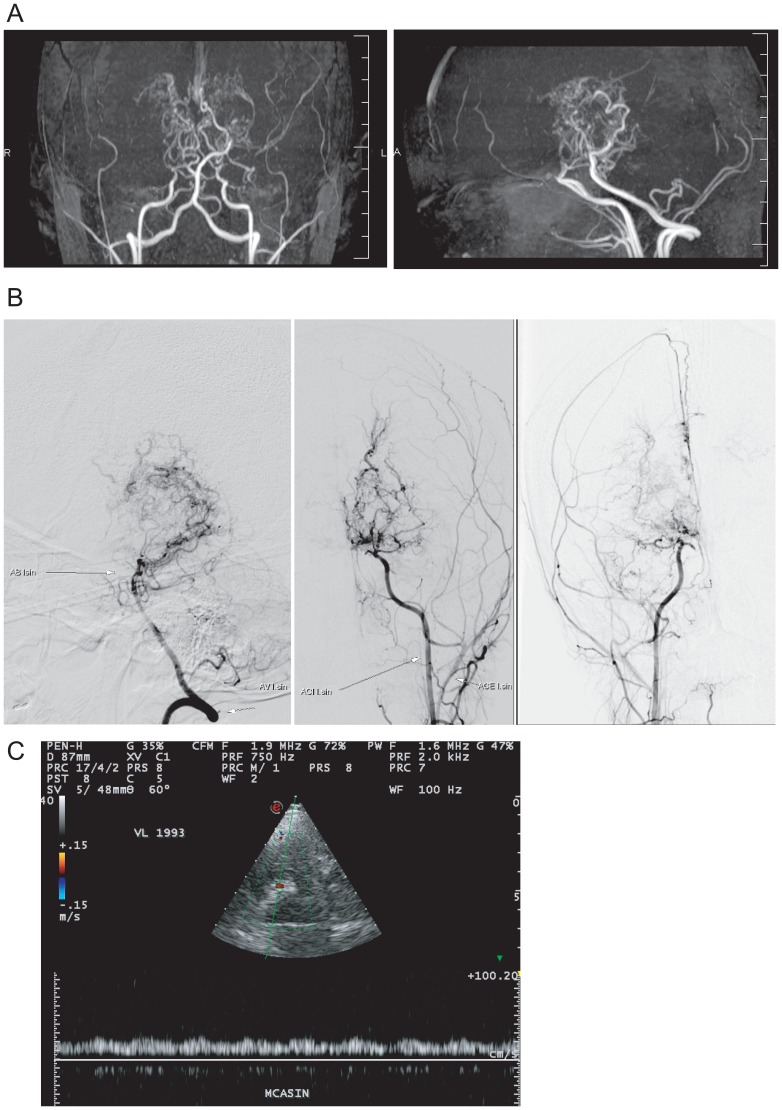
Imaging data of II-2 in Family 1. (A) MRA image. TOF-3D MRA verifies typical steno-occlusive changes of the circle of Willis. Distal T segments of both internal carotid arteries are occluded and basal moyamoya vessels are clearly seen (anteroposterior view, left panel). Typical “puff-of-smoke” look of moyamoya vessels. Internal carotid arteries are relatively hypoplastic compared with the vertebrobasilar system (lateral view, right panel). (B) Digital subtraction angiography. Catheterization angiography of left vertebral artery (left panel), left carotid artery (middle panel), and right carotid artery (right panel). (C) Transcranial color-coded sonography. Severely dampened flow in the M1 segment of the left middle cerebral artery.
